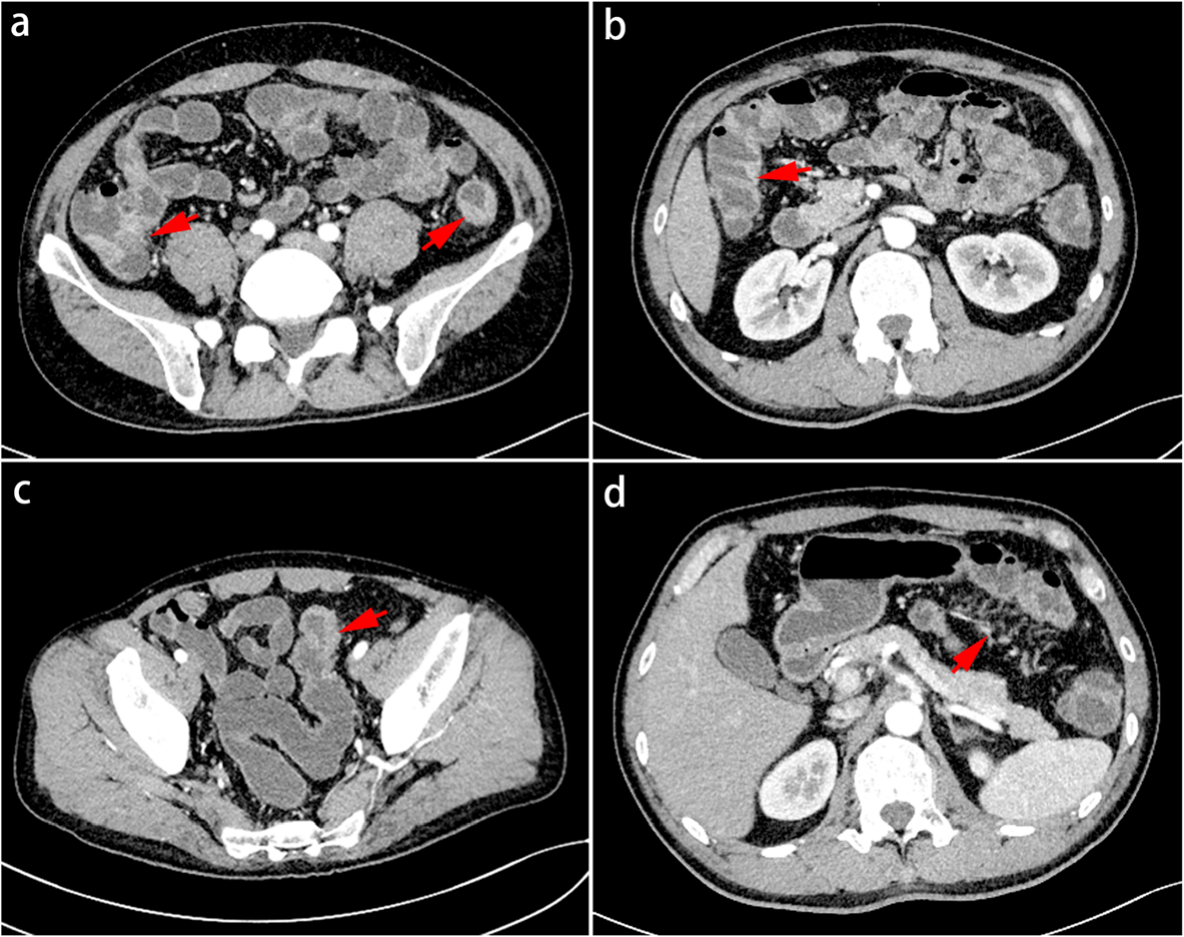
The CT of intestine demonstrated mesentery-side blood vessels around the intestinal loop proliferated, dilated, and twisted. Straight vessels formed comb-like shape. Thickened intestinal wall and lumen mild stenosis were observed from ileocecum to ascending colon, sigmoid colon and the descending colon in turn
